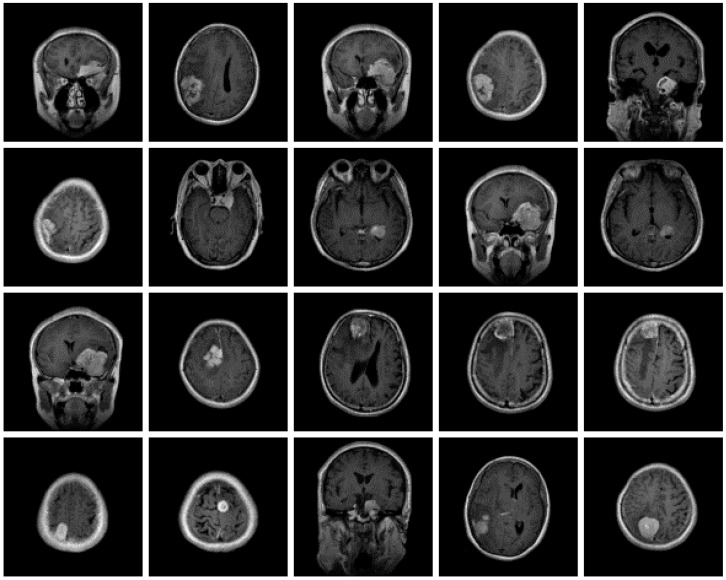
Examples of MRI scans of meningioma tumors.
